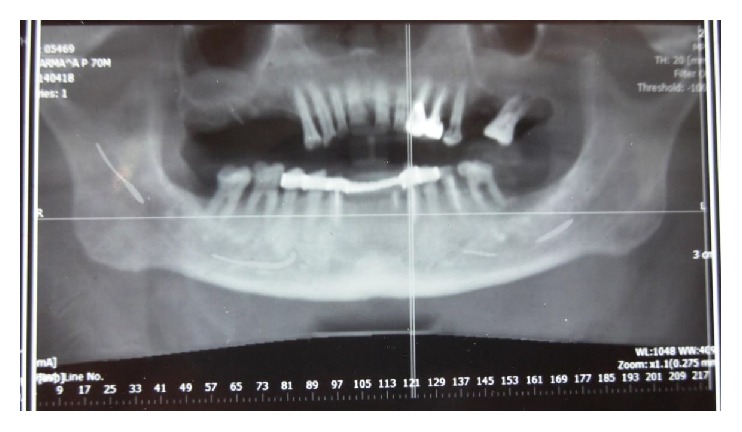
CBCT panorama view reveals heterogeneous soft tissue mass in left retromolar region.
